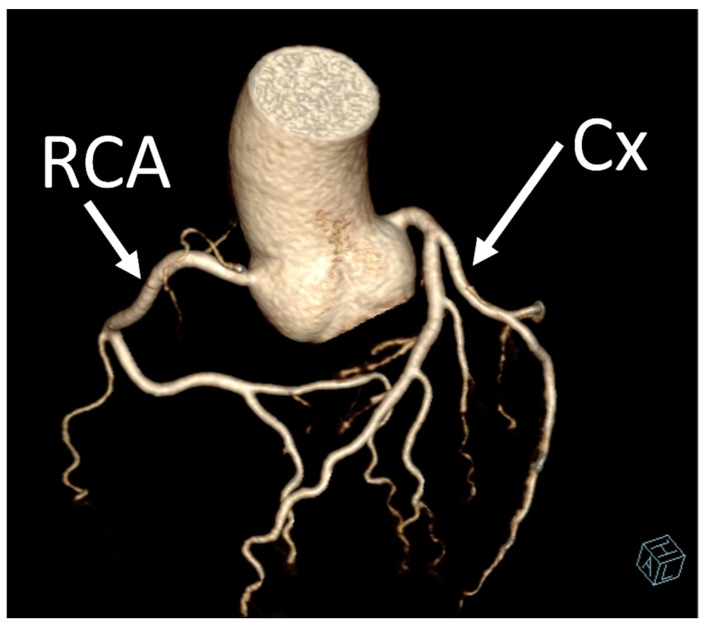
VRT (Volume Rendering Technique) CT image showing the normal origin of the left circumflex artery.
